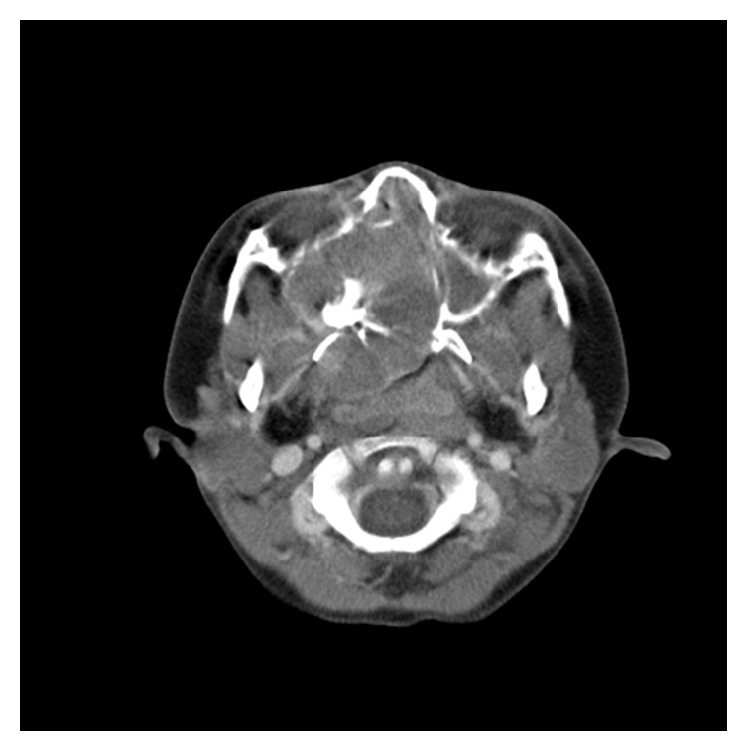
Postcontrast axial CT scan shows various portions of little enhancement within a marked heterogeneous enhancement mass and weaker intensity compared to the facial muscles.
